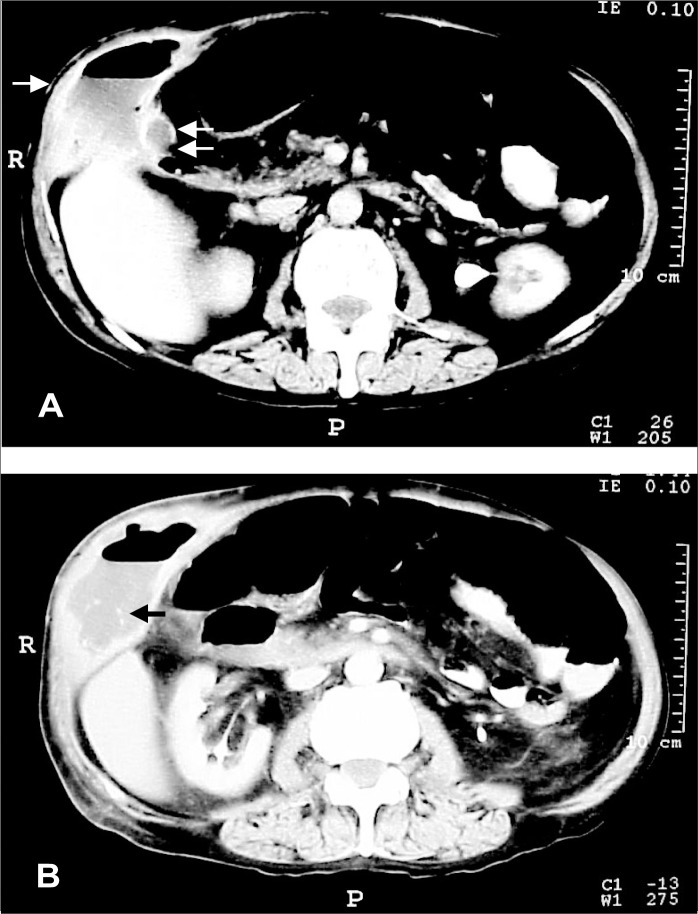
Abdominal computed tomography scan: (A) subcutaneous abscess in the abdominal wall with inner gas (single arrow), and the gallbladder (double arrow); (B) gallstones in the subcutaneous space (arrow).
